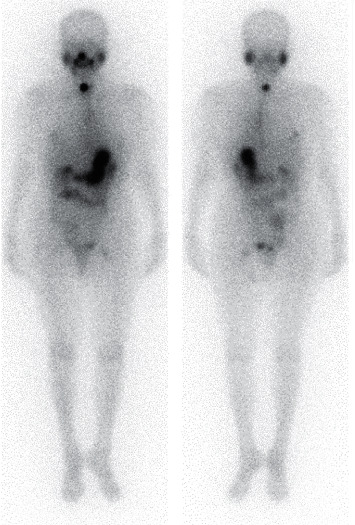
Iodine-131 scintigraphy after radioactive iodine therapy. No uptake was observed in the metastatic lesions.
